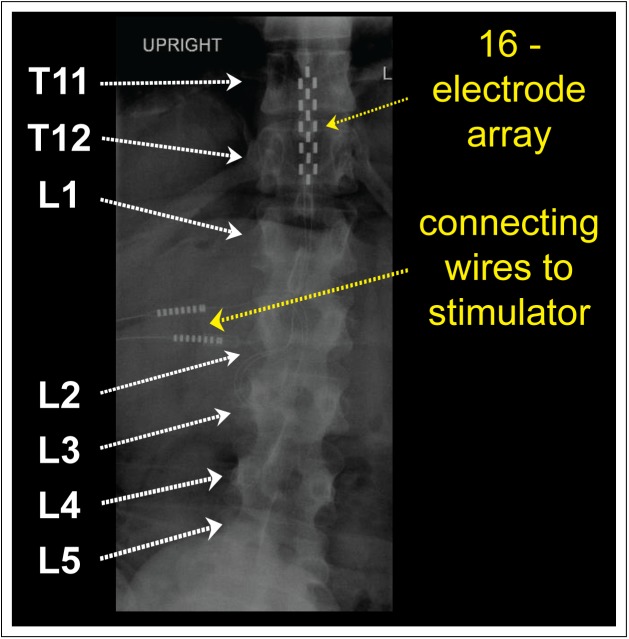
Anatomical placement of 16-electrode array: conventional radiography of the thoracic/lumbar spine displays the position of 16-electrode array (vertebral levels T11–L1).
